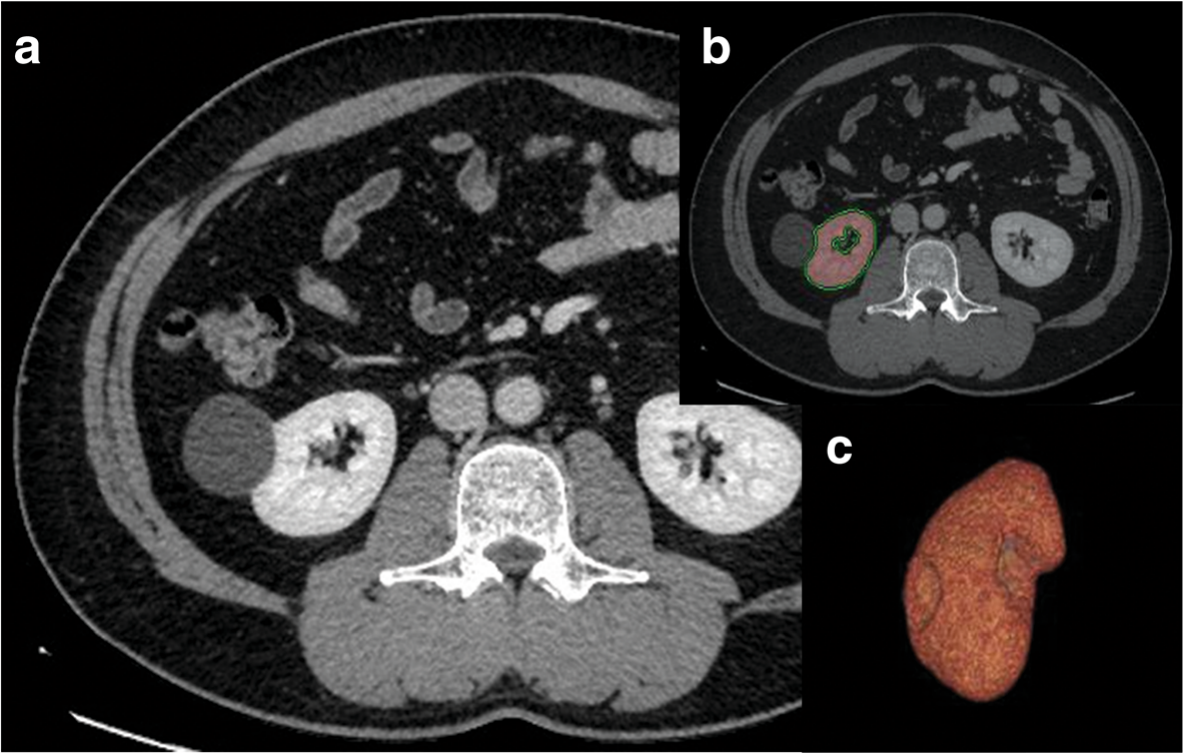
Representative semiautomatic calculation of kidney volume using 3-D reconstruction methods. a An axial plane of the nephrographic phase in a representative patient. b The kidney contour was semiautomatically drawn with pixels of the same CT values being united on an axial plane. c 3-D reconstructed image was created automatically and the volumetry was performed
